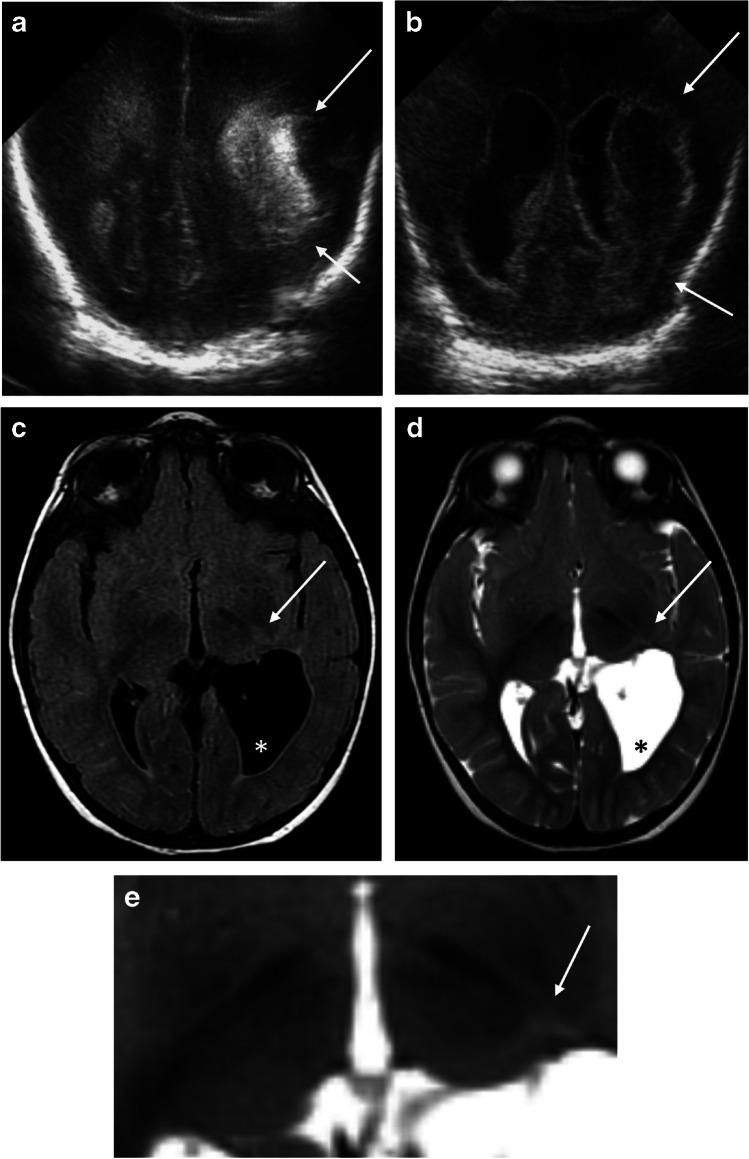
Asymmetric thalamus L-sign in a premature infant girl born at 25 + 6 gestational age (GA). a Brain ultrasound in the coronal plane performed at 5 days of life demonstrates increased echogenicity in the left parietal periventricular and deep white matter (*arrows*), consistent with a left periventricular hemorrhagic infarction. Milder echogenicity in the periventricular and deep white matter is also noted on the right side. b A follow-up brain ultrasound in the coronal plane at 2 weeks of age reveals the evolution of the hemorrhagic infarct (*arrows*) with reduced echogenicity with wider ventricles. c, d Axial fluid-attenuated inversion recovery (c) and T2-weighted (d) brain magnetic resonance images performed at 2 years of age show dilation of the left posterior horn of the lateral ventricle (*asterisks*). This change is secondary to a prior hemorrhage that led to the formation of a porencephalic cyst, which has now become part of the ventricular system. There is more evident white matter volume loss on the left. The thalamus “L-sign” (*arrow*) is only evident on the left. e Magnification of (d), the axial T2 image, again showcases the thalamus “L-sign” (*arrow*)
